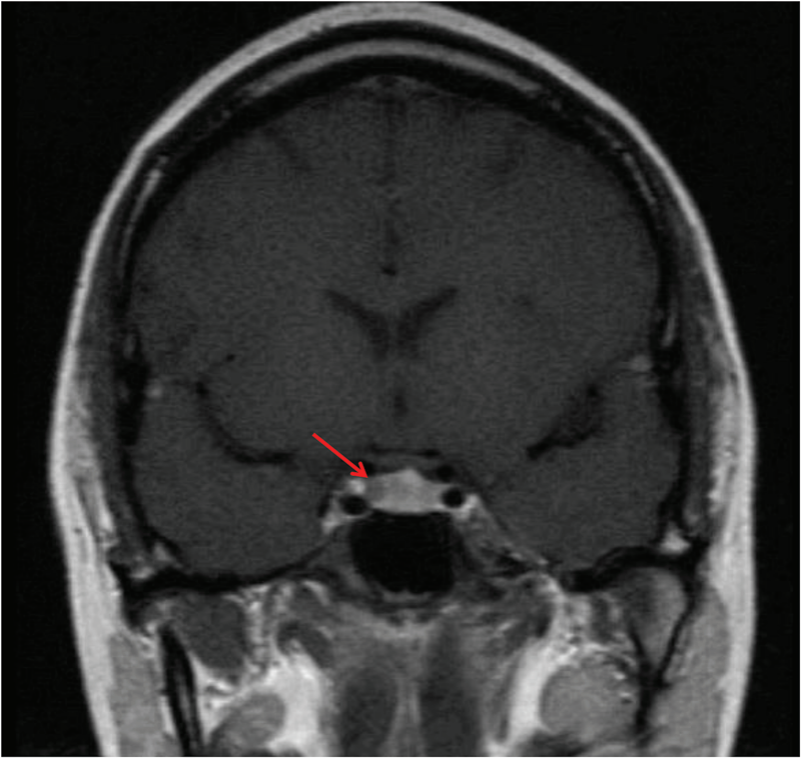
Pituitary magnetic resonance imaging (MRI) coronal image confirmed a right-sided sellar hypointensity consistent with a 4-mm pituitary adenoma.
